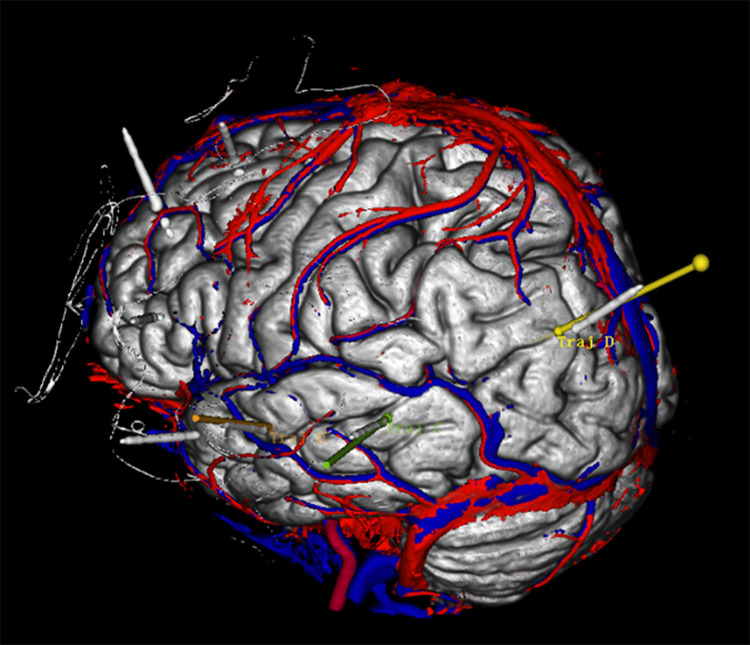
Three-dimensional reconstruction based on CT and MRI images after SEEG electrode implantation. Legend: The target at the tip of electrode B is the hippocampus head, the target at the tip of electrode C is the hippocampus tail, and the target at the tip of electrode D is the amygdala. Additionally, the target in the middle segment of electrode D is the hippocampus body. Fig 5 Cerebral arteries are marked in red, cerebral veins in blue, and the implanted electrodes (B, C, D) are represented by colored cylinders (other SEEG electrodes are shown in gray).
